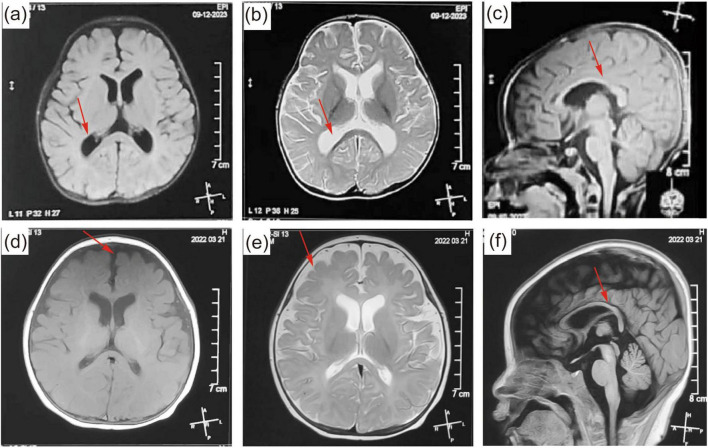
(a–c) Brain magnetic resonance imaging (MRI) of patient 9 at the age of 6 months. The brain MRI showed a widened subarachnoid space, white matter dysplasia, and dysplasia of corpus callosum. (d–f) MRI of patient 13 at the age of 7 months. The brain MRI showed enlarged bilateral ventricles and agenesis of the corpus callosum. The abnormal parts in the brain MRI are pointed out by red arrows.
